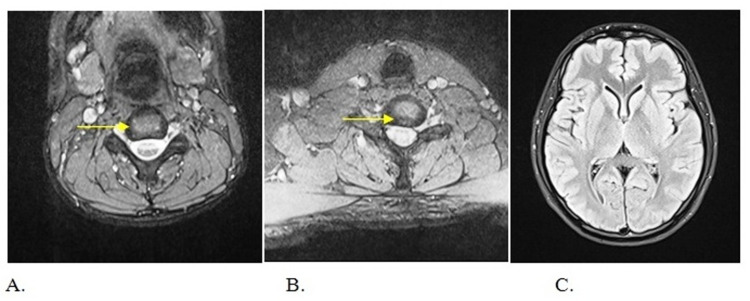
Sequence axial slices demonstrating central signal hyperintensity Sequence axial slices at the level of (A) Cervical spine, (B) Thoracic spine demonstrating central signal hyperintensity (yellow arrow) (C) MRI Brain was normal.
